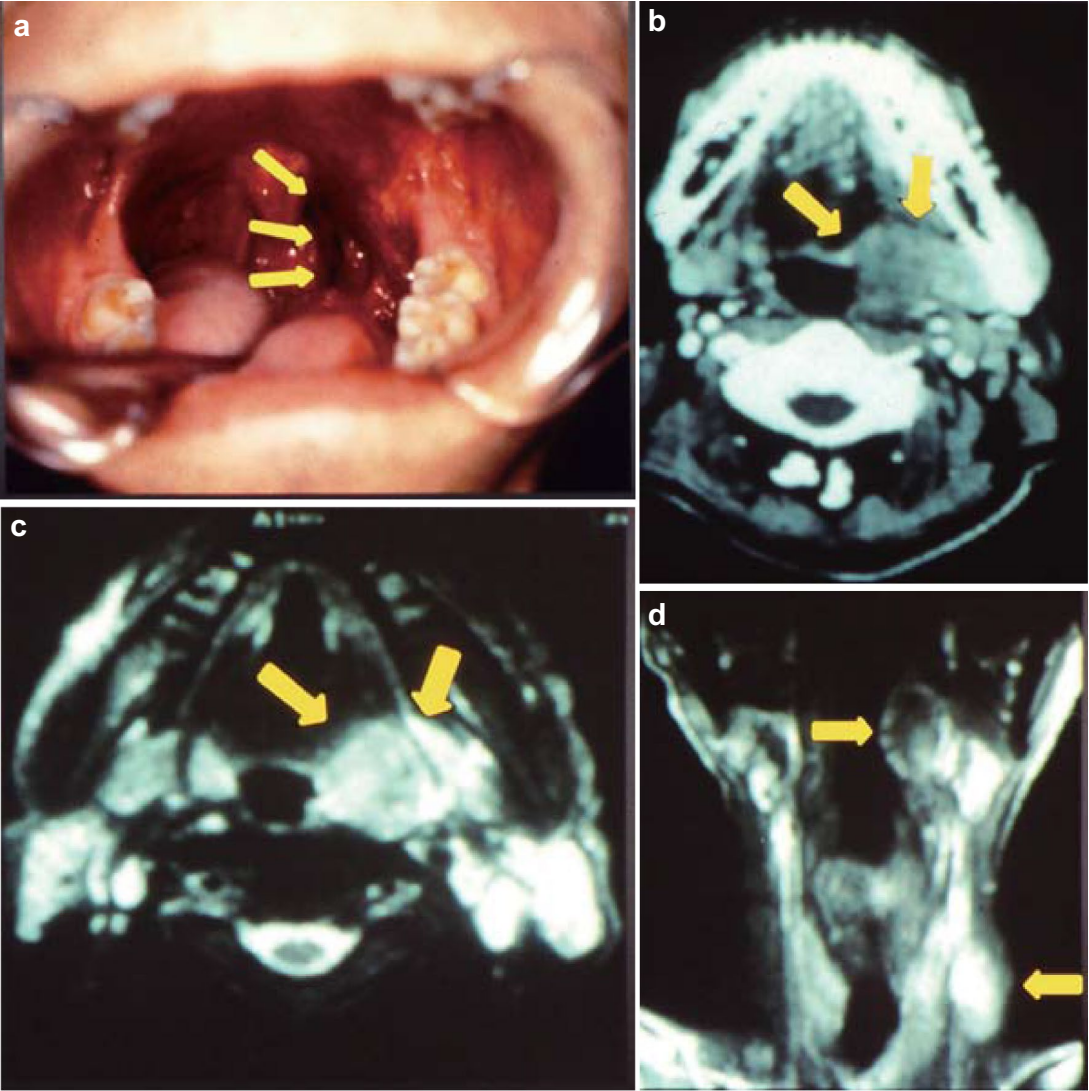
Case 2. a Oropharyngeal image. An ulcerated tumor was observed in the left palatine tonsil (*yellow arrow*). An enhanced tumor was observed in the left palatine tonsil on the contrast-axial computed tomography scan of the neck (*yellow arrow*) (b), contrast-axial magnetic resonance imaging (MRI) scan (*yellow arrow*) (c), and contrast-coronal-MRI scan (*yellow arrow*) (d)
